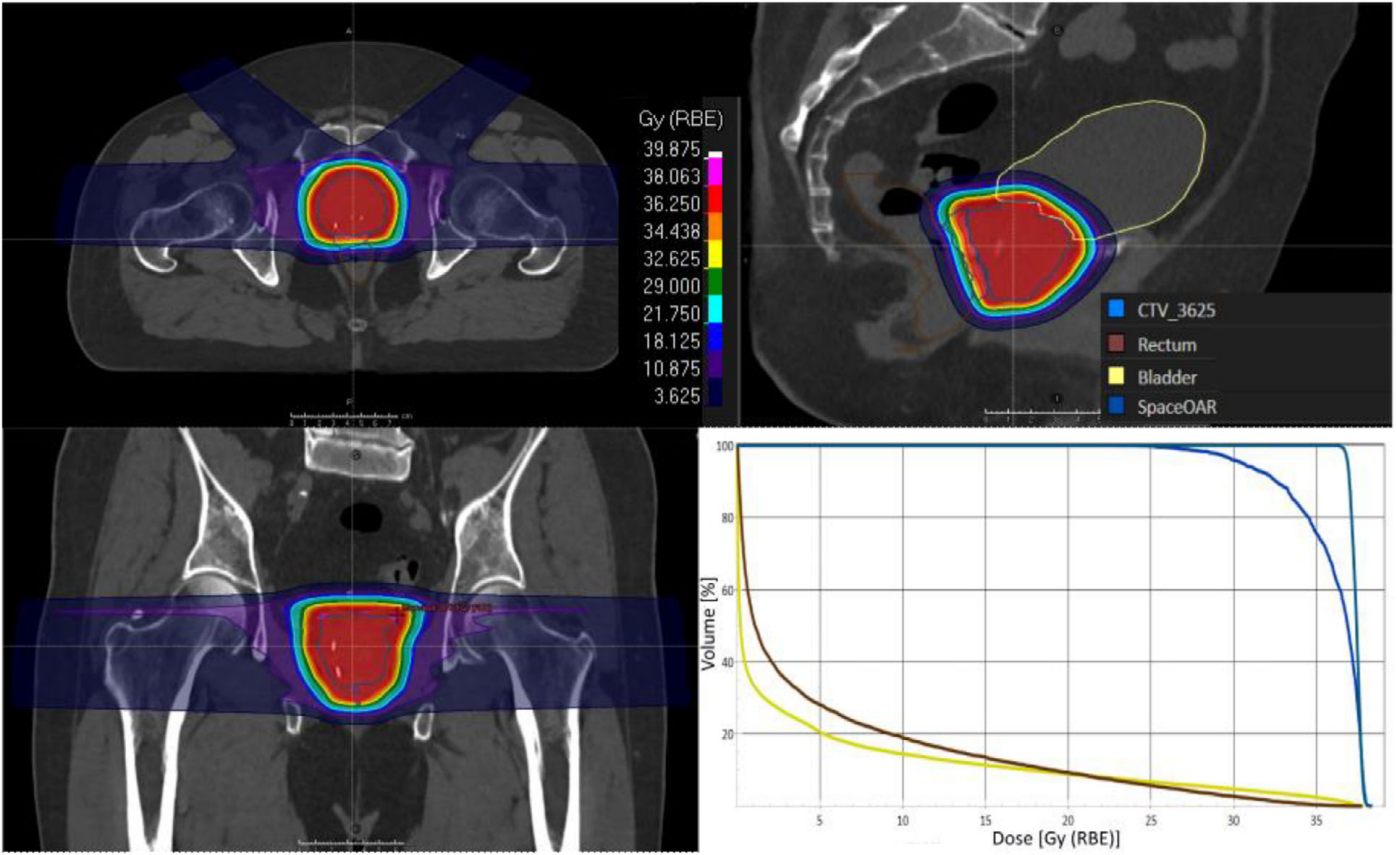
Axial, Coronal, and Sagittal views of dose distribution and the dose volume histogram of a prostate SBRT plan on the initial planning CT showing the target and organs at risk. SBRT, stereotactic body radio therapy.
